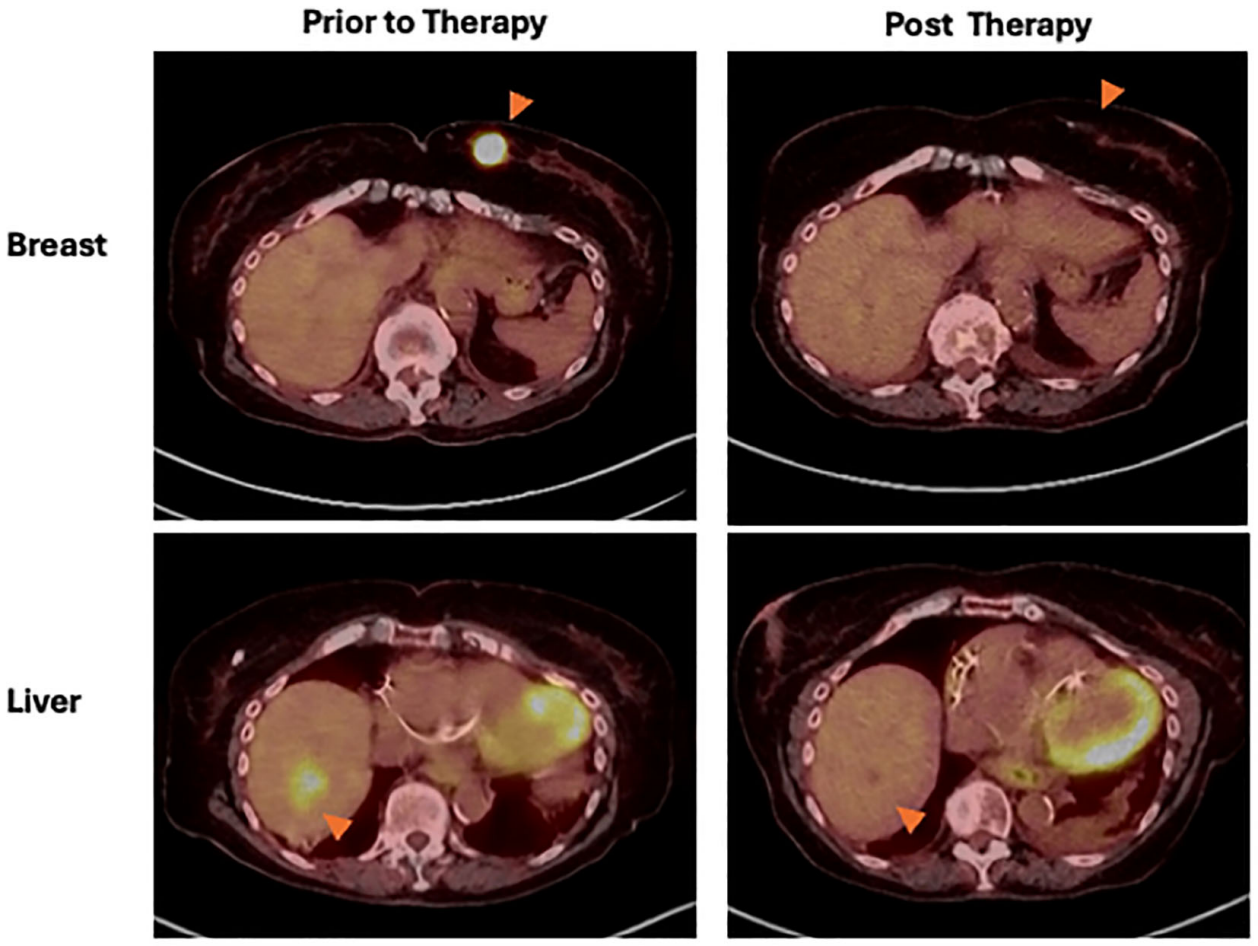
Breast mass (top row) and liver lesion (bottom row) indicated by orange arrowheads. PET CT demonstrates metabolic response of both lesions following pembrolizumab treatment in March 2024 compared to before treatment in September 2023.
